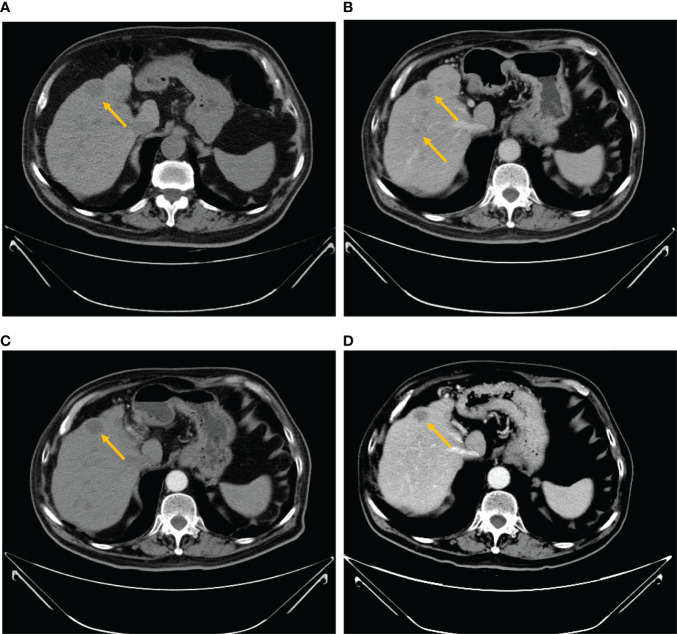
Abdominal computed tomography scan. (A) The baseline CT scan performed on August 26, 2021 showed multiple large hypodense lesions in the liver. Contrast-enhanced CT showed ring-shaped enhanced nodules with the maximum diameter of 17 mm. (B) After 2 months of treatment with imatinib, a CT scan was performed on October 25, 2021, a relapse of disease (growth of the longest lesion’s diameter from 17 to 25 mm) of the hepatic lesion and multiple hepatic metastases with slight reinforcement was observed. (C) The venous phase of the CT scan performed on November 25, 2021 demonstrated decreased hypodense lesions (growth of the longest lesion’s diameter from 25 to 23 mm) with peripheral rim enhancement. (D) After two cycles of sunitinib, a CT scan was performed on January 19, 2022 and revealed a smaller, irregular hypodense intrahepatic metastatic mass.
